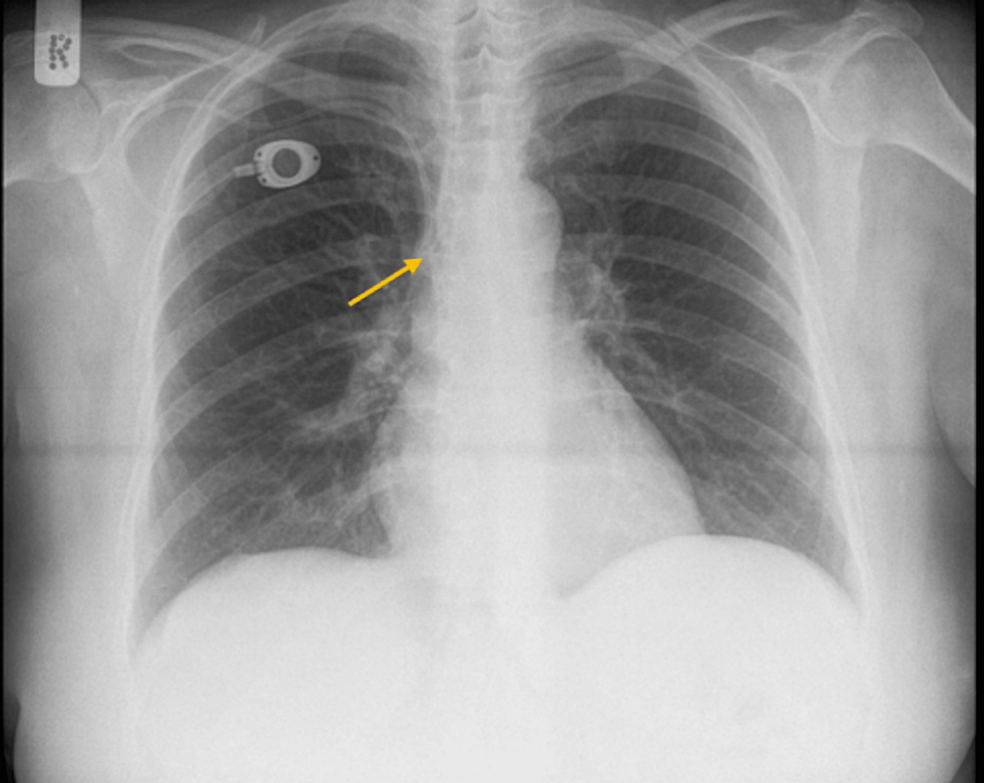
Chest X-ray after the implantation showing the position of the catheter tip in the upper portion of the superior vena cava
